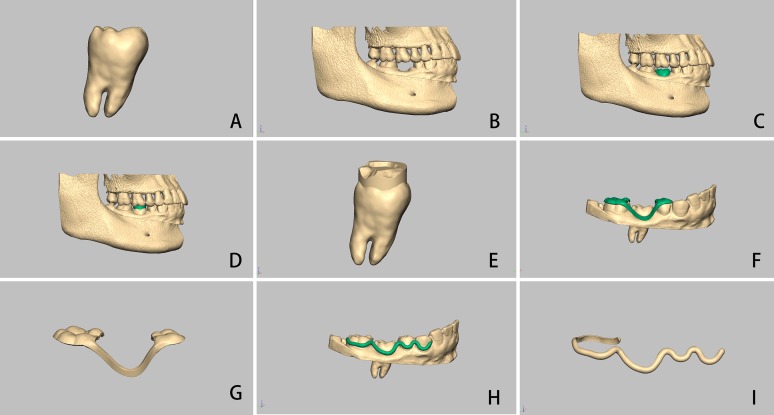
Computer-aided autotransplantation of tooth and virtual design of a series of novel surgical guides. (A) The donor tooth was isolated from CBCT data, (B) digital dentition was registered onto the digital jaw, (C) the digital donor tooth was transplanted onto the recipient site, (D and E) a local surgical split was generated between occlusal surfaces of the donor tooth and its opposite tooth and attached to the donor tooth, (F and G) individual surgical template was supported by adjacent teeth with a connecting bar, (H and I) virtually designed arch bar.
